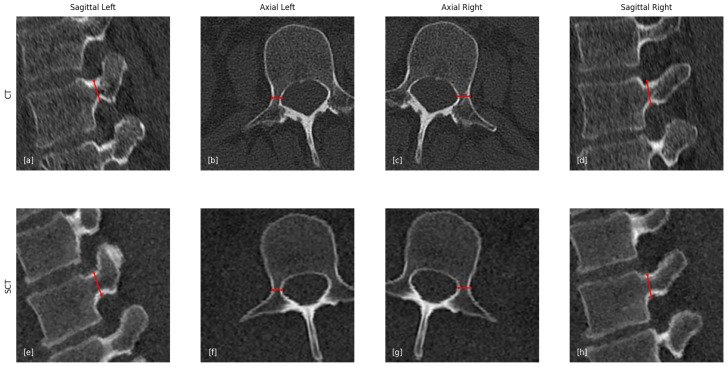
Comparison of the pedicle measurement between CT and sCT. The red line indicate the measurement that was performed. (a,e) CT/sCT sagittal view of left pedicle. (b,f) CT/sCT axial view of left pedicle. (c,g) CT/sCT axial view of right pedicle. (d,h) CT/sCT sagittal view of right pedicle. The red lines present the line that was measured.
